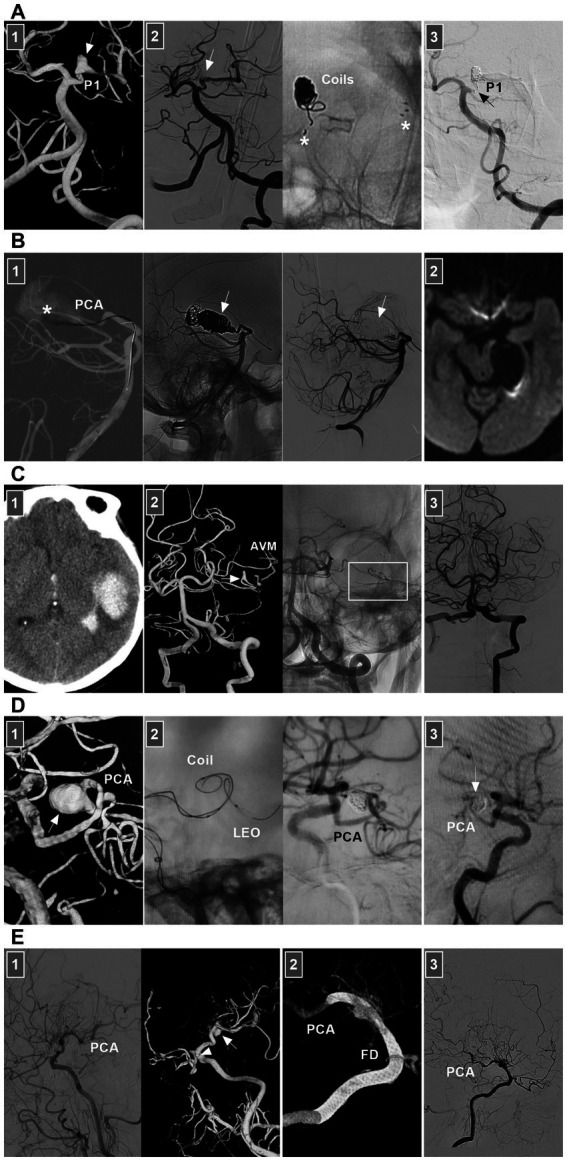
EVT of PCA dissections. (A) Panel 1: DSA showing a P1 dissection (arrow). Panel 2: DSA (left panel) showing that the dissection (arrow) was coiled by stent assistance, and X-ray image (right panel) showing the coils and stent (asterisks). Panel 3: Six-month follow-up DSA showing that the P1 segment of the PCA was nearly occluded (arrow). (B) Panel 1: X-ray image (left panel) showing the microcatheter went into the giant distal PCA dissection aneurysm (asterisk) to wait for the coiling, DSA and unsubtracted DSA (middle and right panels) images showing the aneurysm (arrows) and the parent PCA were occluded. Panel 2: Diffuse weighted image of magnetic resonance showing no ischemia of PCA region. (C) Panel 1: CT showing left temporal hemorrhage. Panel 2: DSA (left panel) showing an AVM supplied by the branch of PCA with a flow-related dissecting aneurysm (arrow), and unsubtracted DSA (right panel) showing the dissection was embolized by casting Onyx (frame). Panel 3: DSA showing the AVM was obliterated. (D) Panel 1: DSA showing a sidewall dissecting aneurysm (arrow) of distal PCA. Panel 2: X-ray image (left panel) showing the design of the EVT by LEO stent assisted-coiling, and DSA (right panel) showing the aneurysm was embolized. Panel 3: Six-month follow-up DSA showing the aneurysm (arrow) had no recurrence. (E) Panel 1: DSA (left panel) showing the moyamoya disease and well-developed PCA, and DSA (right panel) showing a distal PCA dissecting aneurysm and an ophthalmic aneurysm (arrowhead). Panel 2: Vaso-reconstructive image showing that the FD covered two aneurysms. Panel 3: DSA showing the PCA was patent. AVM, arteriovenous malformation; CT, computed tomography; DSA, digital subtraction angiography; EVT, endovascular treatment; FD, flow diverter; PCA, posterior cerebral artery; P1, first segment of PCA.
